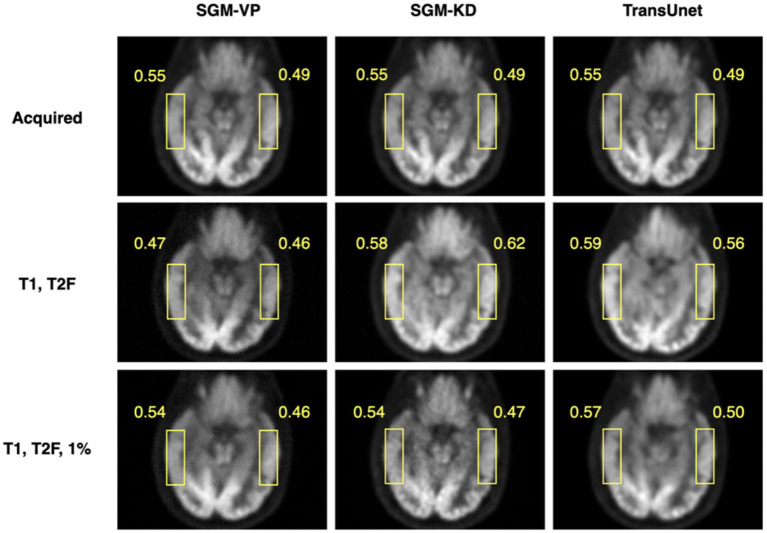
Axial slice for one case with asymmetrically lower metabolism in the left temporal lobe based on the clinical report. We computed the mean SUVR in the left and right yellow boxes with values shown on top. We observe better agreement with the acquired PET for ultralow-dose task compared to zero-dose task.
